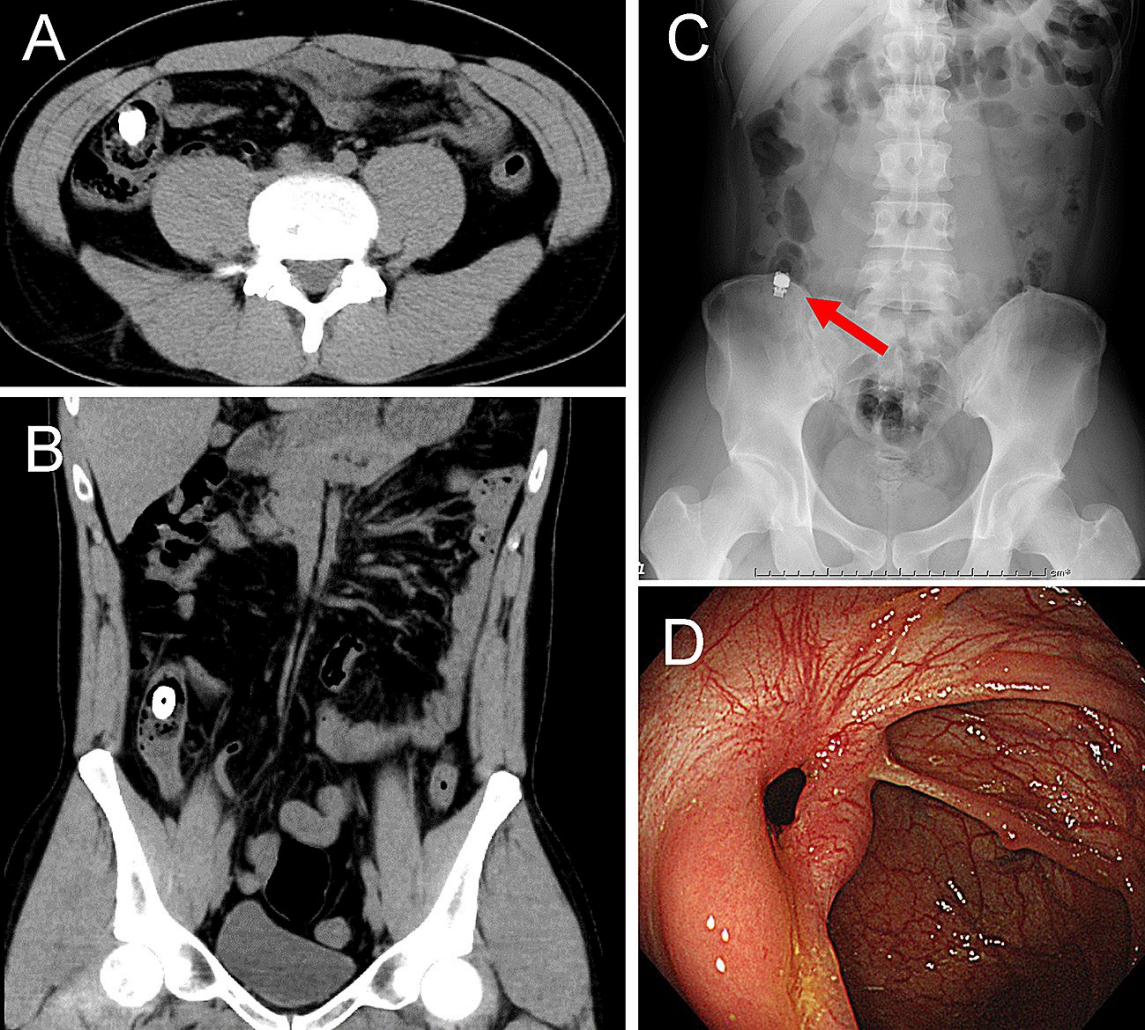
SBCE retention case caused by CT misjudgement of PC in a patient with Crohn’s disease. Plain computed tomography (CT) images show that the PC seemed to be in the ascending colon; axial CT image (A), coronal CT image (B). X-ray scan confirms SBCE retention (arrow) in the terminal ileum after 2 weeks (C). Colonoscopic image confirms ileocecal valve stenosis, which was treated with balloon dilatation (D).
